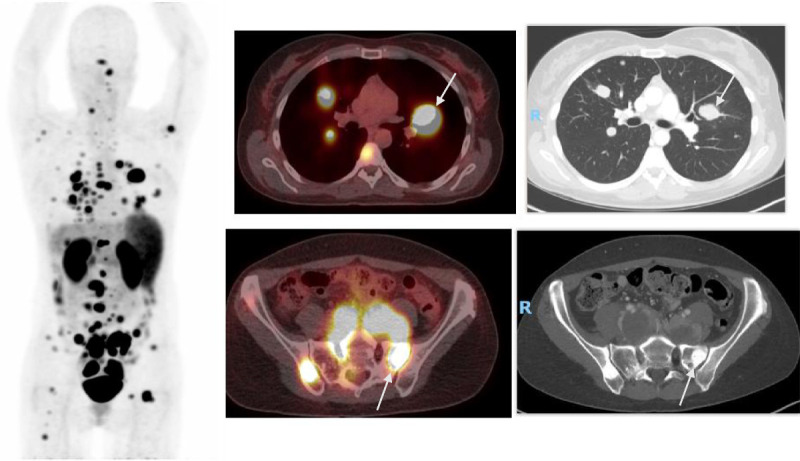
Major change: treatment with PRRT. Multiple ^68^Ga-DOTATOC avid lung lesions, extensive lymphadenopathy, and multiple sclerotic bone lesions on whole body and fused PET-CT and CT images. Representative transaxial CT slices show bilateral lung nodules and bilateral sclerotic iliac bone lesions (arrows on representative lesions) with intense uptake (SUVmax up to 29.5) on fused PET-CT image at the same level. Major change recommendation: PRRT.
